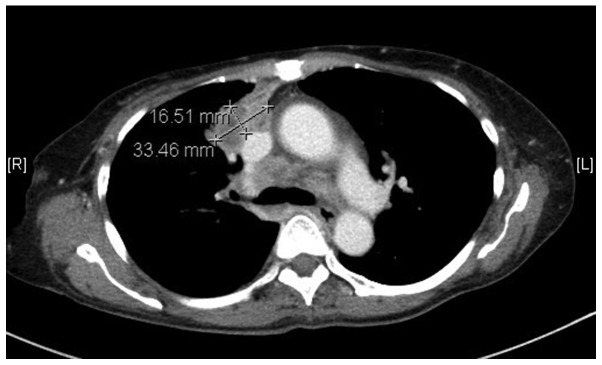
Computed tomography scan of the thorax showing a large lobulated mass in the right upper lobe, measuring 3.3×1.6 cm.
